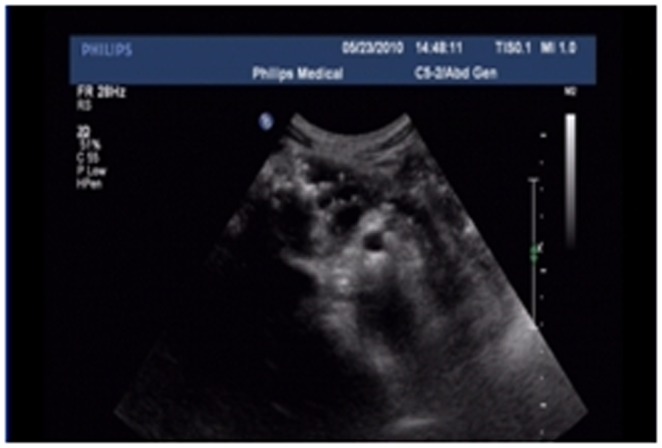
Color Doppler ultrasonography showing string-of-beads dilation of pancreatic duct.
